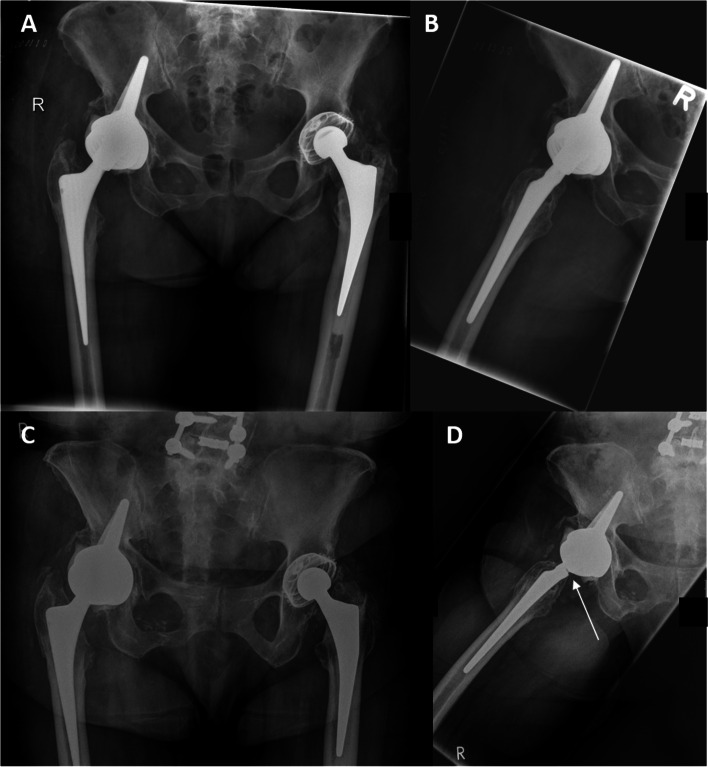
(A + B) Postoperative radiographs after double socket procedure with a dual mobility cup (DMC) implanted in a socket cup (09/2016). The taper shows no signs of damage at this stage (B). At FU (03/2020; C + D), the cup position had remained unchanged, however, signs of advanced taper corrosion were seen on the lateral radiograph (D), indicated by the groove at dorsal aspect of the cone (arrow), presumably due to taper impingement on the dorsal aspect of the DMC
